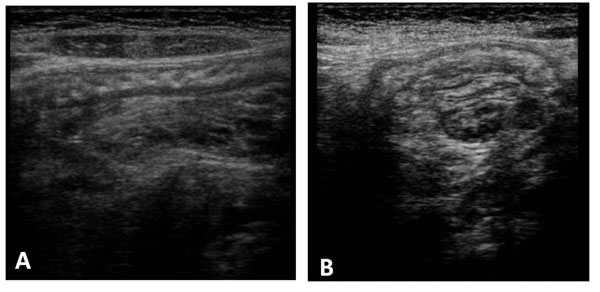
Ultrasound features of intussusceptions. On the longitudinal scan obtained at the centre of the mass show the sandwich sign formed by three parallel hypo-echoic bands separated by two nearly parallel hyper-echoic bands. The outer hypo-echoic band represent the edematous everted limb of the intussuscepted and the thin intussusceptions, the central hypo-echoic band is the central limb of the intussuscepted. The hyper-echoic bands are caused by the mesentery that is dragged along with the bowel loop. Us scan obtained at the apex show the hair-fork-sign form by three parallel hypo- echoic bands (the prongs of the hair fork), separated by two hyper-echoic bands formed by mesentery. (a) Us scan obtained at the base shows the central limb of the intussusceptions eccentrically surround by the hyper-echoic mesentery that show the crescent in doughnut sign. (b)
